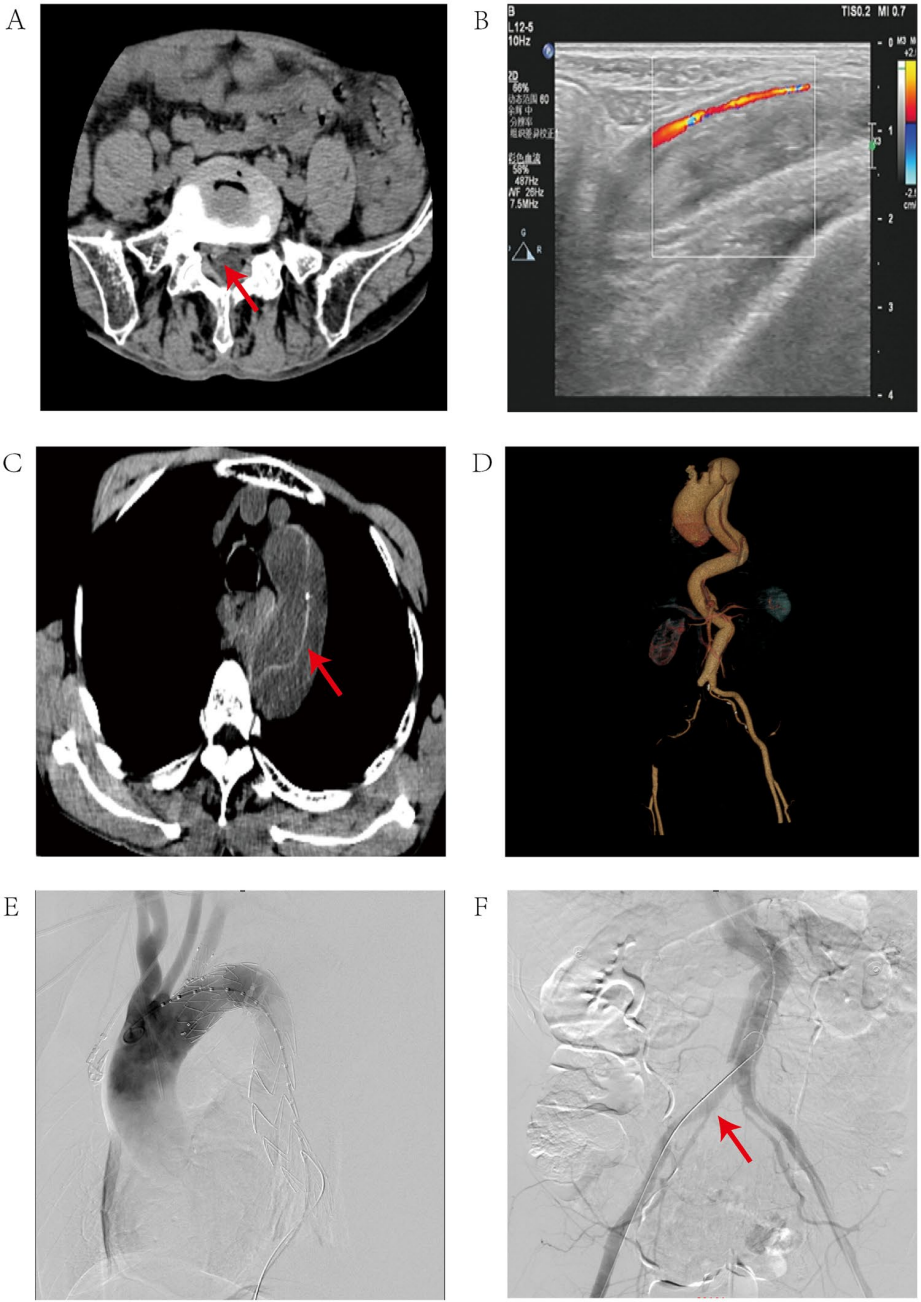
A Axial CT showing right posterior nucleus pulposus protrusion (arrow). B Vascular ultrasound demonstrating near-complete right common iliac artery occlusion (white box). C Axial CTA revealing aortic dissection flap (arrow). D 3D CTA reconstruction showing dissection extent and occluded right iliac artery (arrow). E Angiogram poststent placement. F Poststenting angiogram confirming iliac artery patency (arrow)
